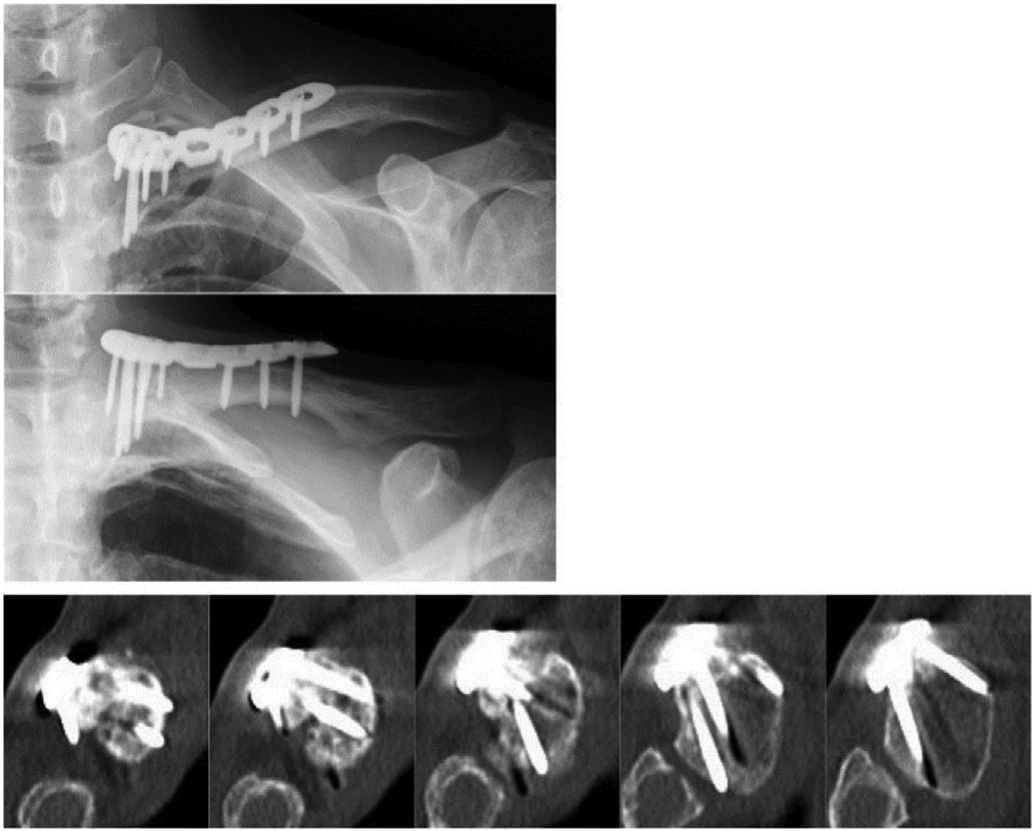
One-year follow-up radiographs demonstrating union of the fracture without loosening of the internal fixation. A computed tomography scan showed that all 2.7-mm locking head screws had been precisely and safely inserted into the medial fragment.
